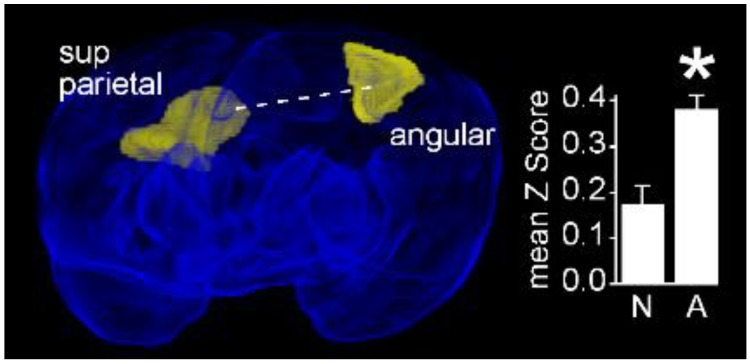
The left angular gyrus exhibits a decreased functional connectivity in infants with normal movement character compared with infants with jerky movement character. Obliquely projected 3D reconstructions of MRI images of cerebral grey matter and identified parcellated regions derived from a neonatal atlas. Dashed white line depicts the connectivity relationship. Inset: Fisher-transformed Z-scores ± 1 SEM. N: Normal; A: Abnormal. * *P*_FDR_ < 0.05.
